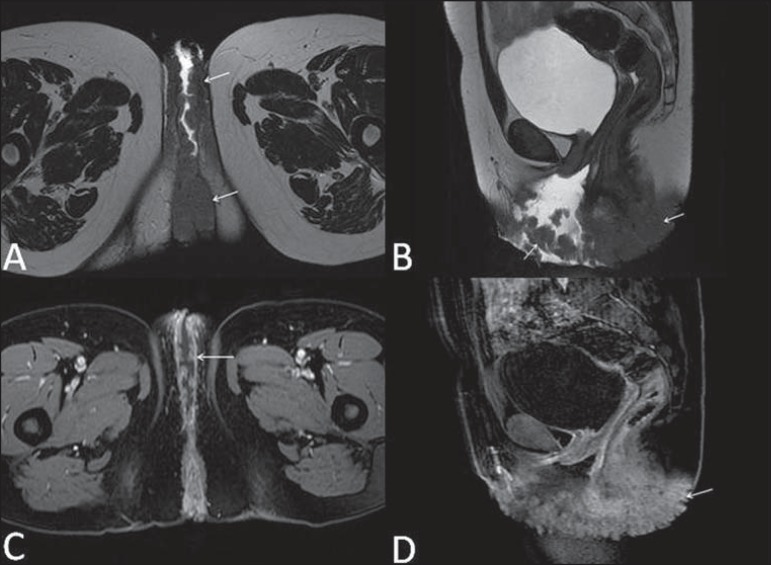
Giant condyloma acuminatum. Contrast-enhanced, multiplanar MRI T2- weighted
